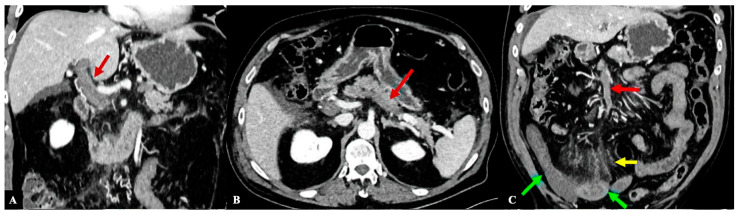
Panels (A–C) show massive spleno-mesenteric-portal thrombosis and intestinal ischemia by computed tomography (CT) imaging at time of hospital admission. (A) Coronal CT scan in the post-contrast venous phase showing complete thrombosis of the portal vein (red arrow). (B) Axial CT scan in the post-contrast venous phase showing complete thrombosis of the splenic vein (red arrow). (C) Coronal CT scan in the post-contrast venous phase showing complete thrombosis of the superior mesenteric vein (red arrow). The intestinal loops, especially on the right side, showed thickening and hypoperfusion of the walls with trilaminar appearance (“target’’ sign—indicated by green arrows) and edema of mesenteric adipose tissue (yellow arrow).
